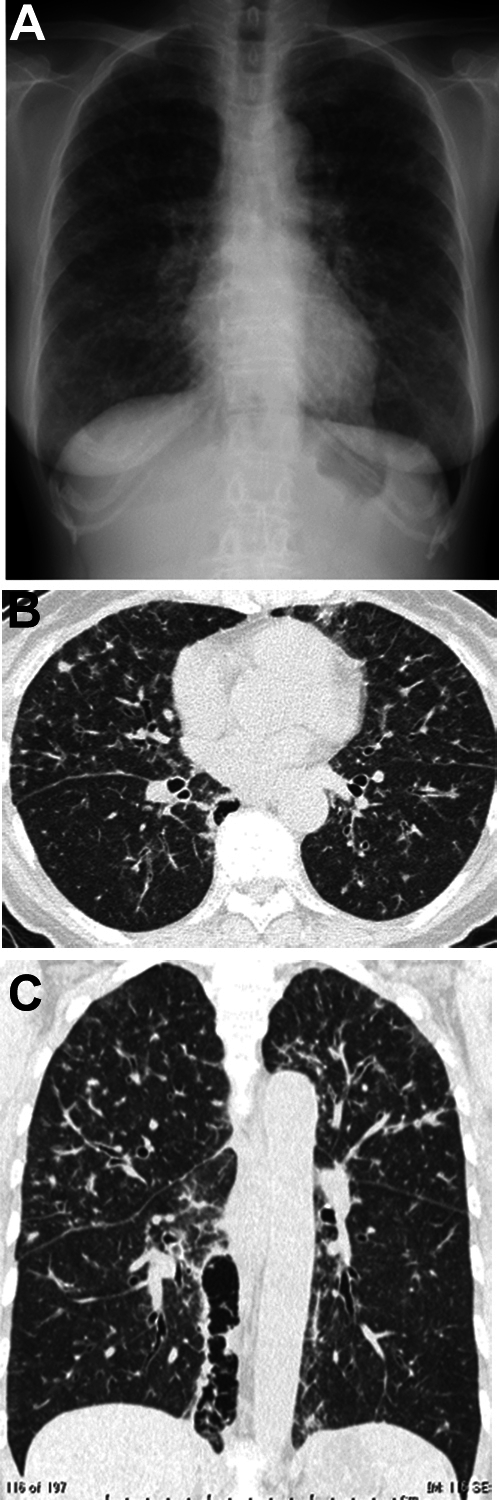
A-C, Chest imaging obtained during the patient’s first visit to the hospital. A, Chest radiographs showing diffuse ground-glass opacities and small nodules in the bilateral lung fields. B, C, Chest CT scans—axial view (B) and coronal view (C)—showing diffuse bilateral thickened bronchovascular bundles, multifocal ground-glass opacities, and small nodules with perilymphatic distribution. Interlobular septal thickening and a thin-walled cavity in the right lower lobe adjacent to the mediastinum measuring 37 × 22 × 100 mm also are present.
